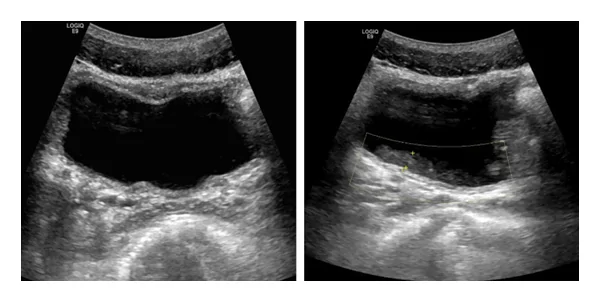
Ultrasound of the bladder after cessation period, demonstrating improvement.
